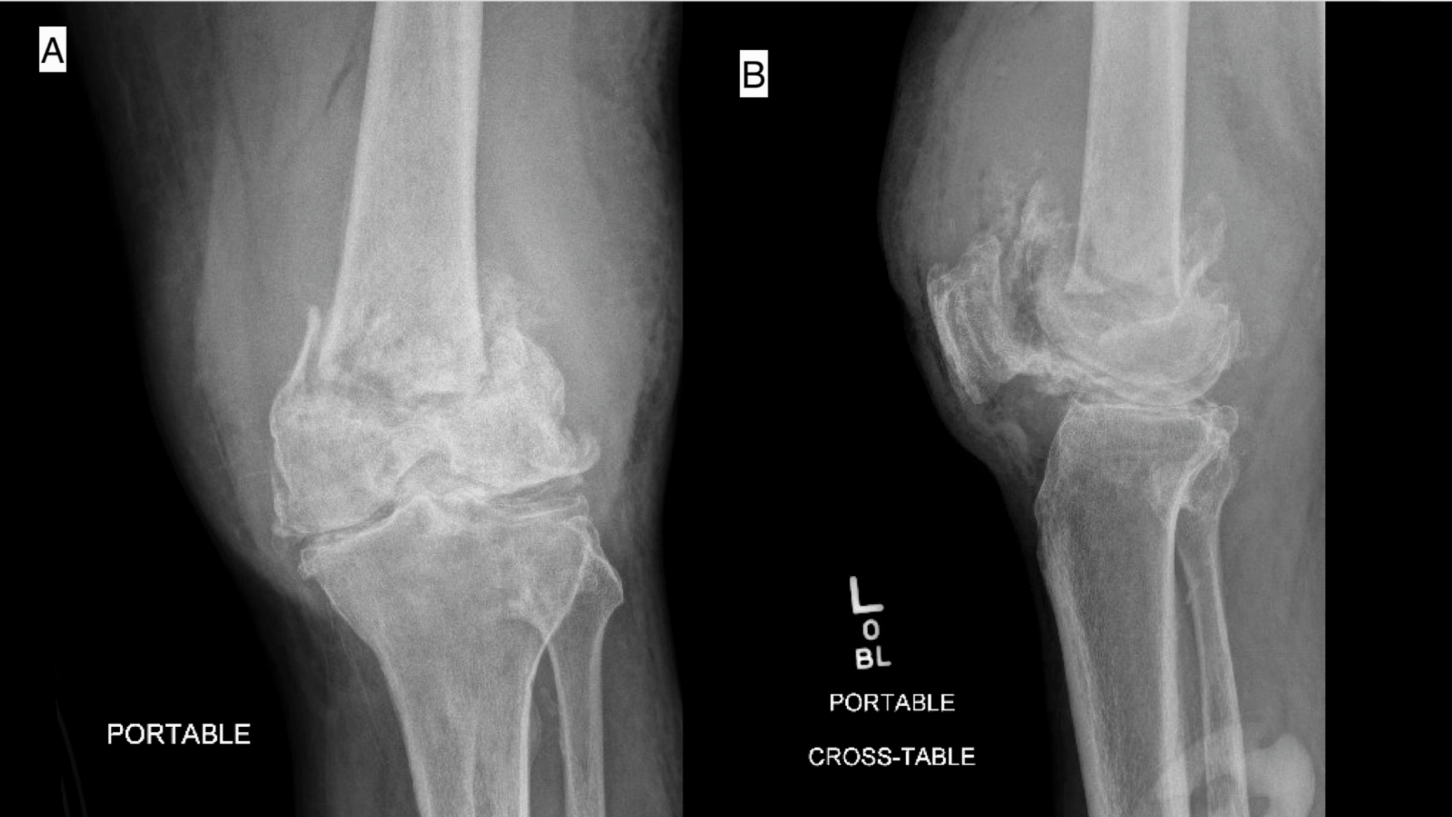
Knee (A) anteroposterior and (B) lateral radiographs taken immediately after aborted total knee arthroplasty.
